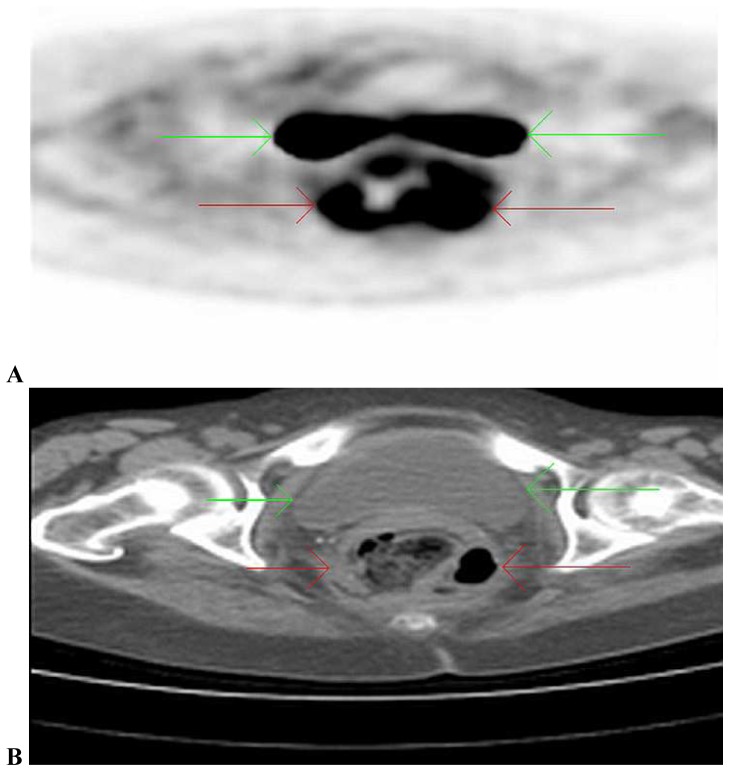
Axial PET/CT images showing physiological 18-FDG uptake in the bladder (green arrow) and increased peri-rectal 18-FDG uptake (A, red arrow). Additionally, the presence of air is detected on the low-dose CT (B). This was not present on an abdominal CT-scan that was performed prior to the PET/CT-scan. A diagnosis of peri-rectal abscess was confirmed during explorative surgery.
